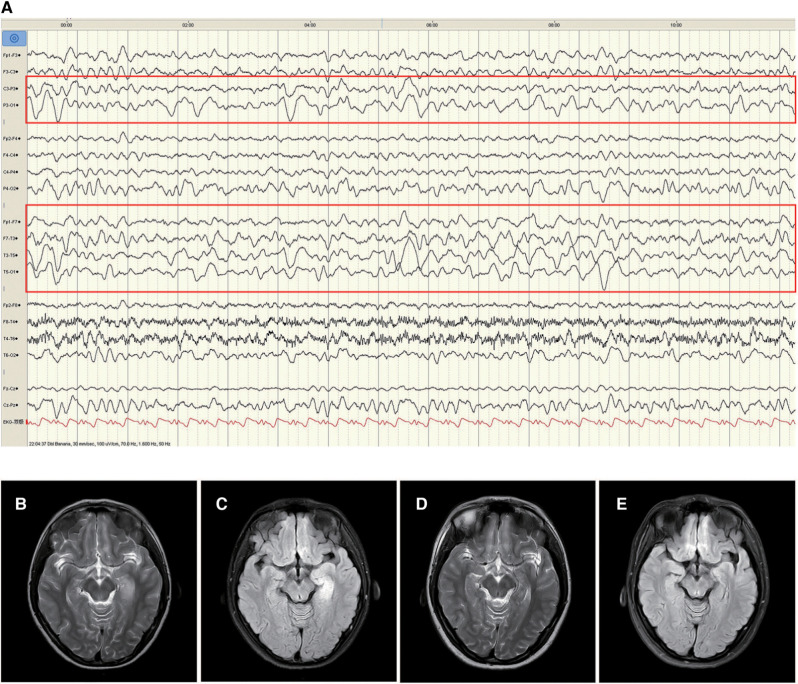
Electroencephalogram (EEG) and changes in brain magnetic resonance imaging (MRI) of patient 1. The left temporo-occipital polymorphic *δ* and *θ* activities were observed in the EEG of the patient in the waking phase at disease onset (A, red rectangle). MRI T2/fluid-attenuated inversion recovery (FLAIR) hyperintensities in the hippocampal gyrus in the left medial temporal lobe (B and C). Improvement of abnormal signal in MRI T2/FLAIR (D and E) three years after treatment.
